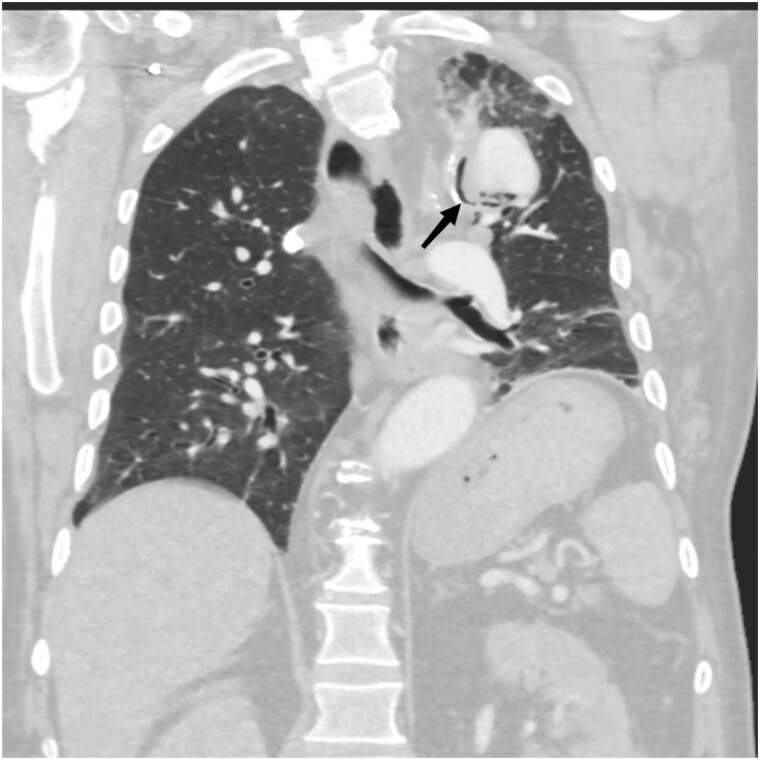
CT angiography in the parenchymal window shows a prosthetic fistula involving the lung.
